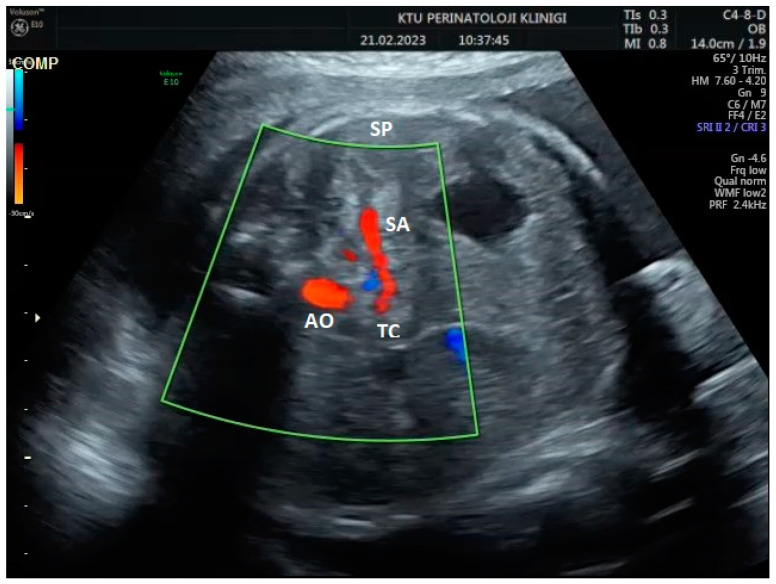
Two-dimensional ultrasonographic imaging in the axial plane of the 30-week-old fetus shows the origin of the fetal splenic artery from truncus coeliacus (TC; truncus coeliacus, AO; aorta, SA; splenic artery, SP; fetal spleen).
